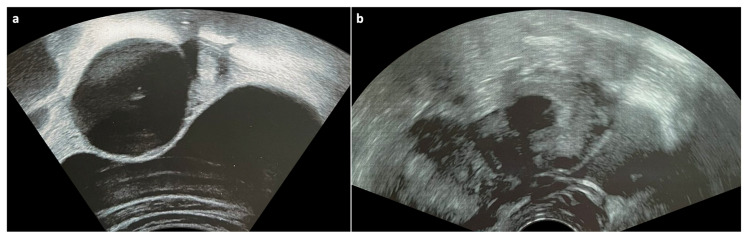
Ultrasonograms of two adolescents demonstrating (a) a thin-walled follicular cyst with posterior acoustic enhancement and (b) a thick-walled corpus luteum cyst.
